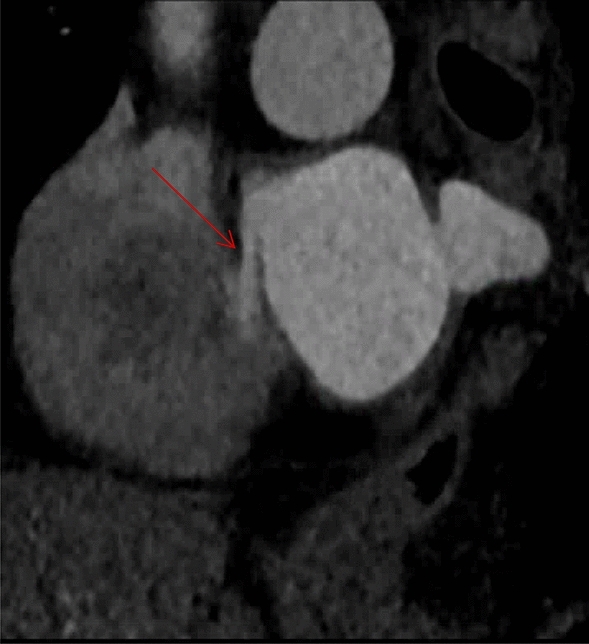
Oblique sagittal reformation showing a crypt-shaped contrast jet from the left atrium to the right atrium toward the vena cava (red arrow) on cardiac CT, indicative of a PFO which was later confirmed on TTE with agitated saline contrast
